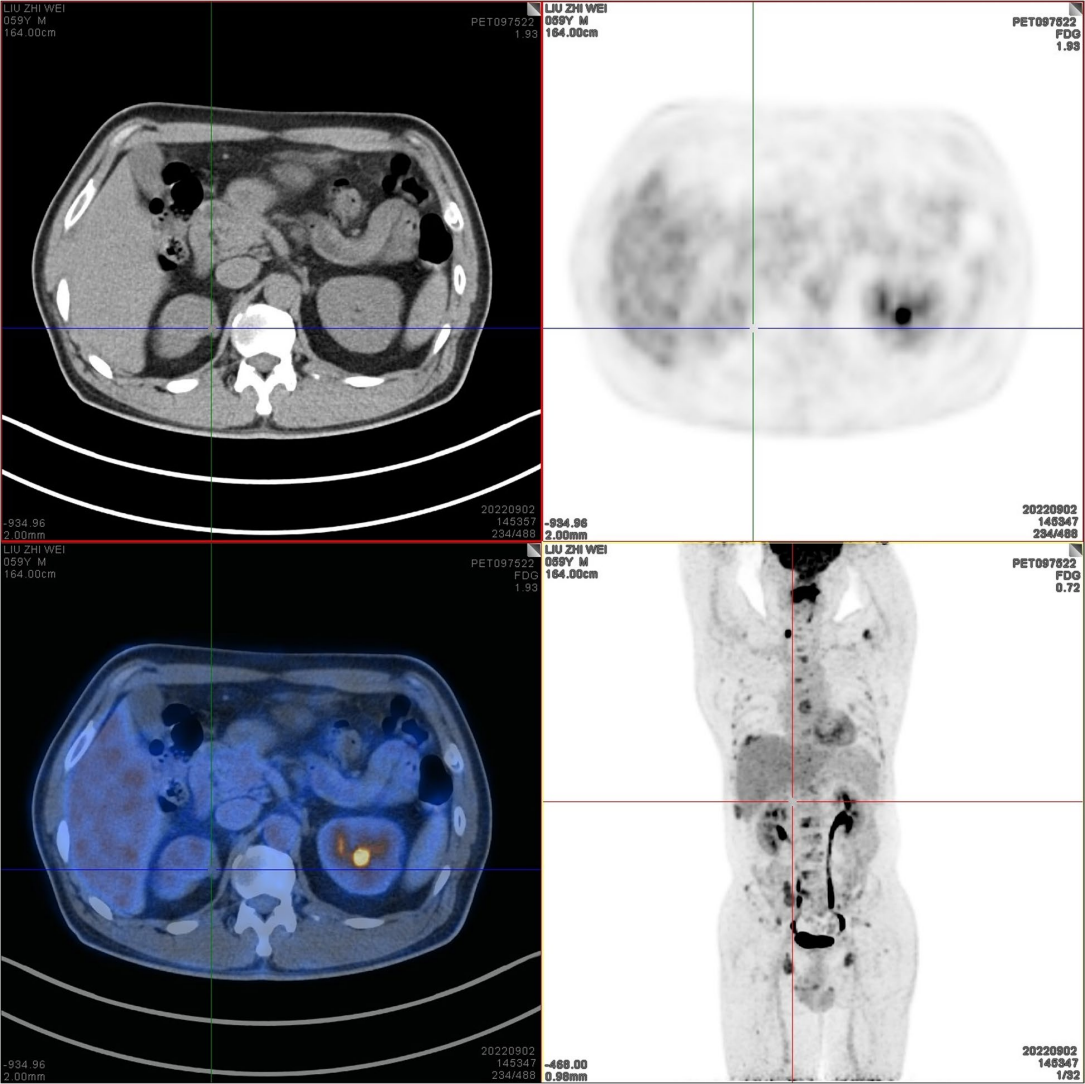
PET-CT scan findings of an isodense nodule in the right superior renal pole, approximately 25 mm in diameter
